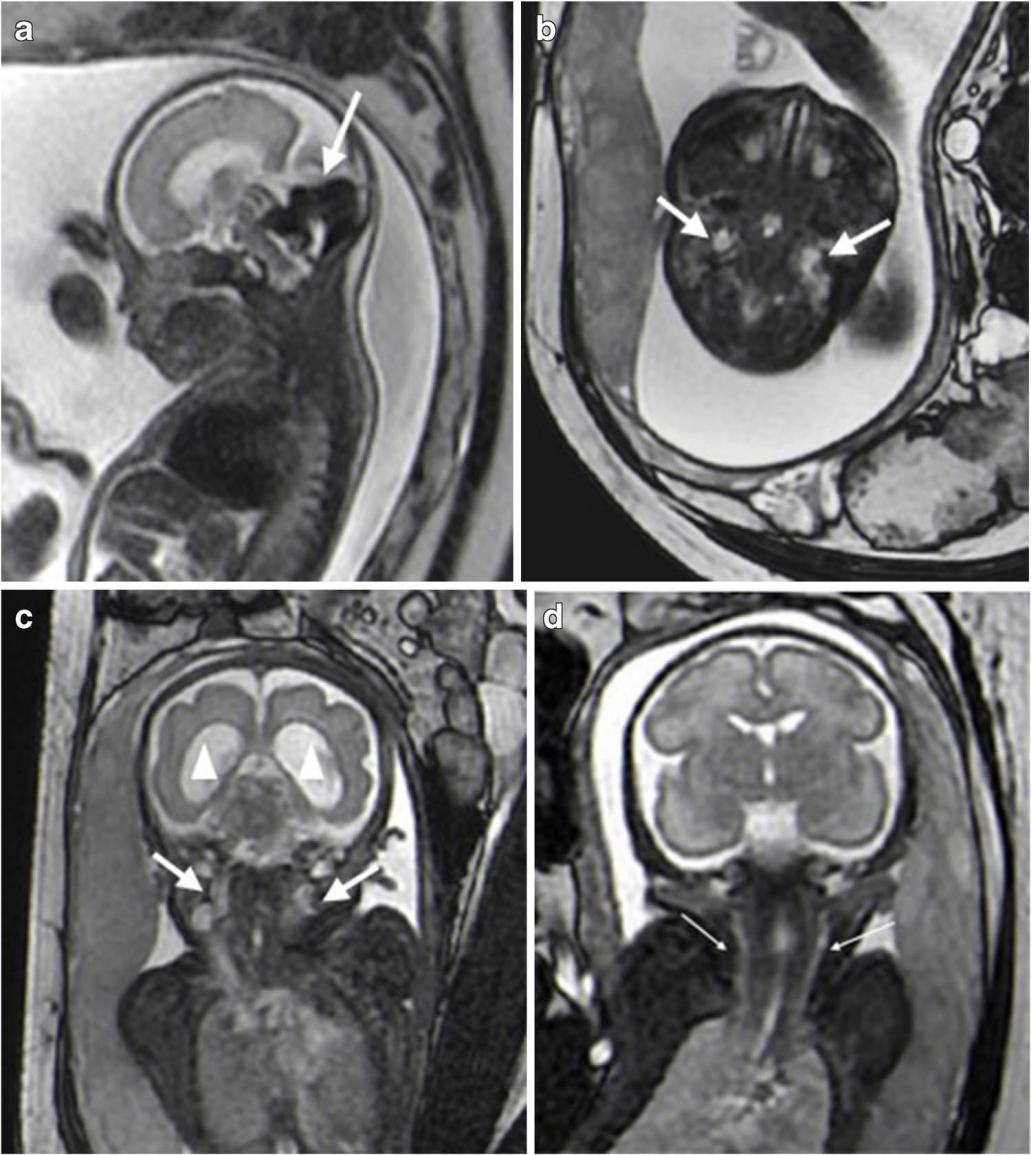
Magnetic resonance imaging in a 28-week-old female fetus with a vein of Galen aneurysm. a A sagittal single shot fast spin echo image shows the vein of Galen dilatation (*arrow*). b An axial balanced steady-state free precession (B-SSFP) image shows bilateral internal jugular vein dilatation at the level of the atlantoaxial joint (*arrows*). c A coronal B-SSFP image shows bilateral internal jugular vein dilatation (*arrows*) and lateral ventricle enlargement (*arrowheads*). d A coronal B-SSFP image of a normal fetus at 28 weeks of gestation with normal bilateral internal jugular veins (*arrows*)
